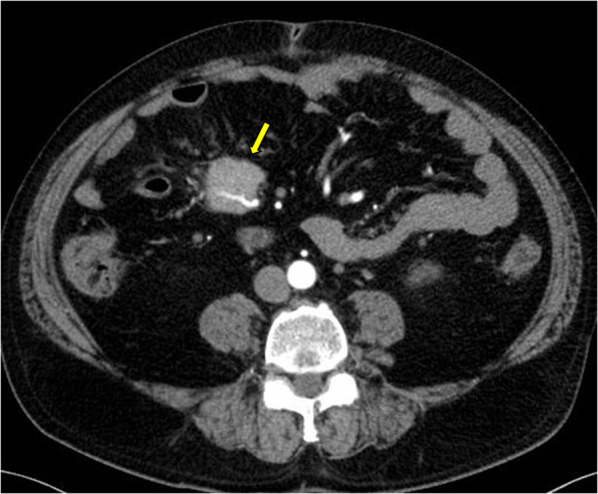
Contrast-enhanced computed tomography of the abdomen reveals a mesenteric lobulated mass with an artery running through the center (arrow)
